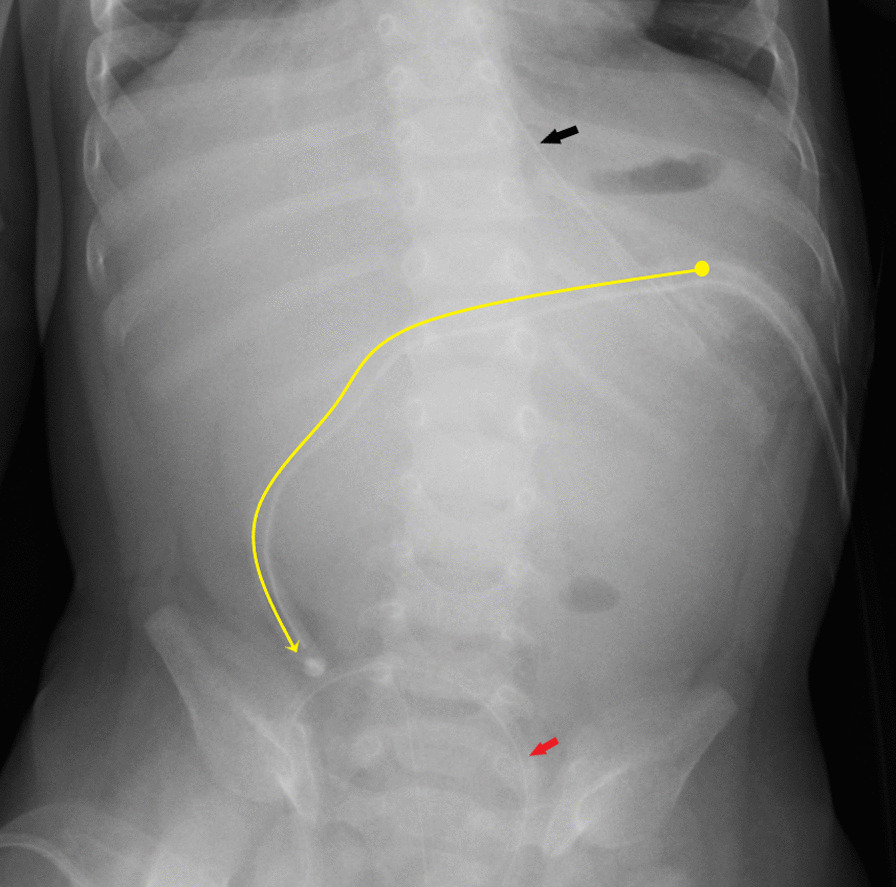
Upright abdominal X-ray the Foley catheter 1 month after Pezzer catheter replacement. The intraabdominal part of the Foley catheter is indicated (yellow line). Nasogastric tube (black arrow) and bladder catheter (red arrow)
